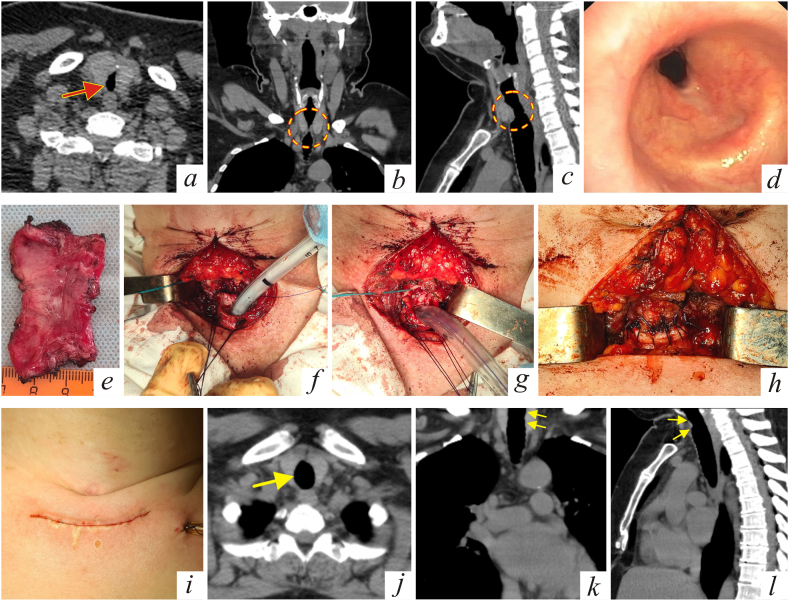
Typical patient (female, 50 y.o.) from our cohort who developed post-resuscitation CTS after COVID-19 associated pneumonia: pre-op (*a*) axial, (*b*) coronal, and (*c*) sagittal computed tomography (CT) scans and (*d*) bronchoscopic image showing tracheal lesion; (e*-i*) intra-op images exemplifying the excised tracheal fragment and surgical stages of end-to-end anastomosis formation according to Fig. 1; (*j-l*) post-op CT scans in six months, where yellow arrows indicate the consistent anastomotic area. (For interpretation of the references to colour in this figure legend, the reader is referred to the Web version of this article.)
